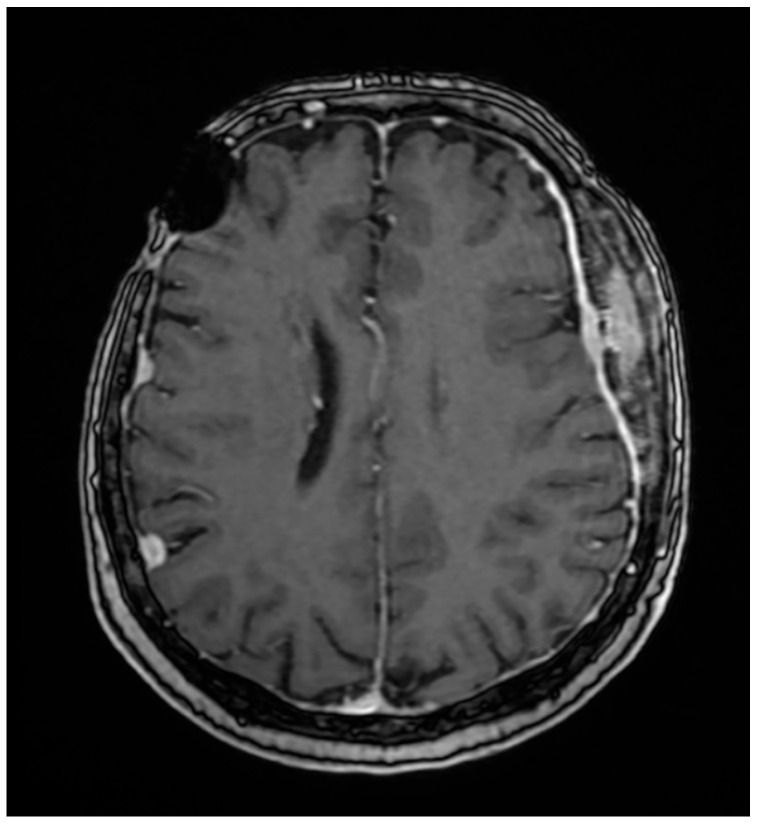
MRI examination with iv contrast media, axial 3D T1 FSPGR (14 February 2023). MRI (2023) showing a porencephalic cavity in the left fronto-temporo-parietal region, post-neurosurgical intervention. Tumor-related changes include a large central osteolytic lesion (49 × 22 × 76 mm) with irregular bone structure and soft tissue extension. Surrounding inhomogeneous osteosclerosis, diffuse meningeal thickening, and mild temporal muscle edema are noted. No contrast enhancement is seen in the porencephalic cavity. Stable multiple meningiomas are also present.
